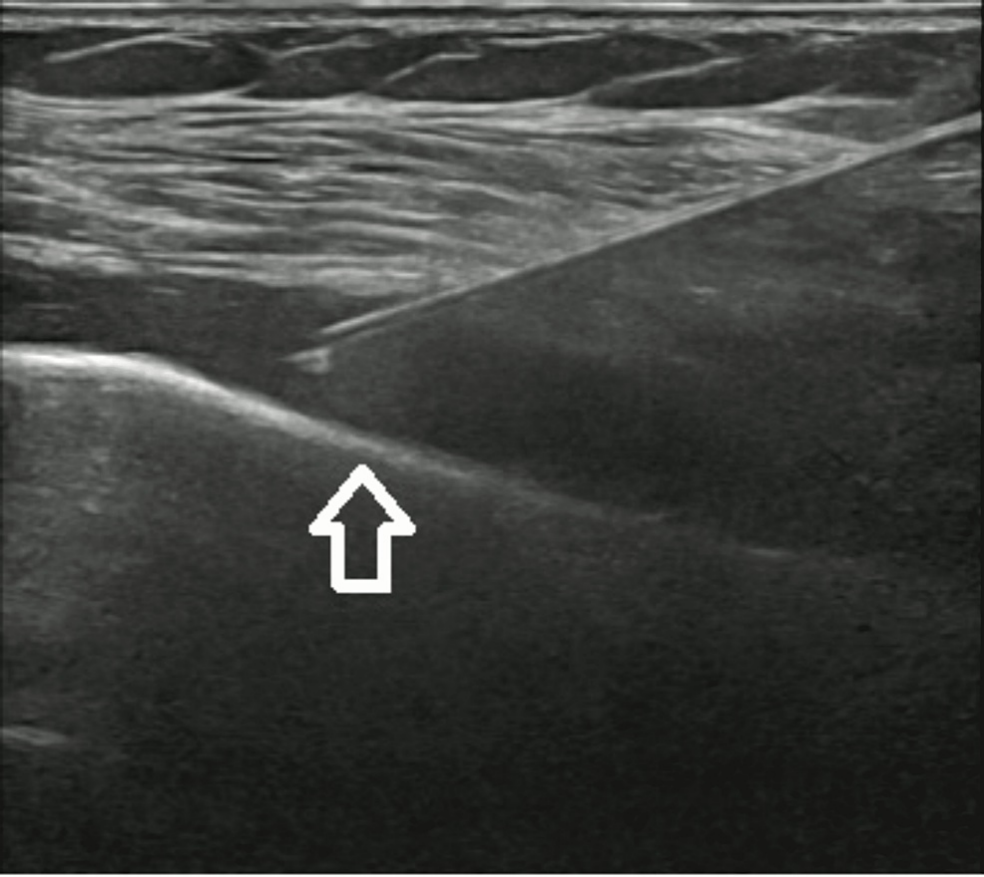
Ultrasound-guided left shoulder fluid drainage.
